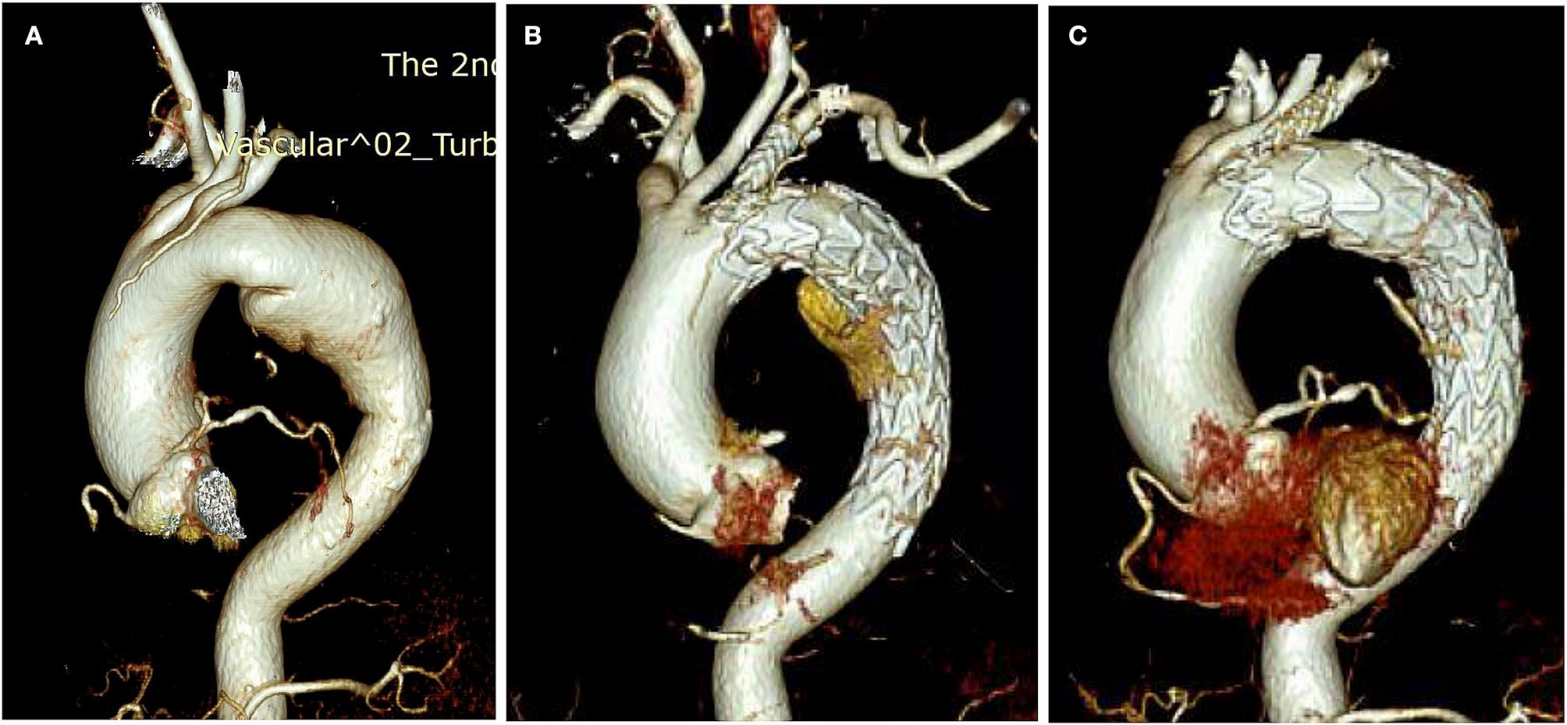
The CTA of the aorta before and after the TEVAR. (A) Before TEVAR; (B) 1-week CTA check after TEVAR; (C) 6 months CTA check after TEVAR.
